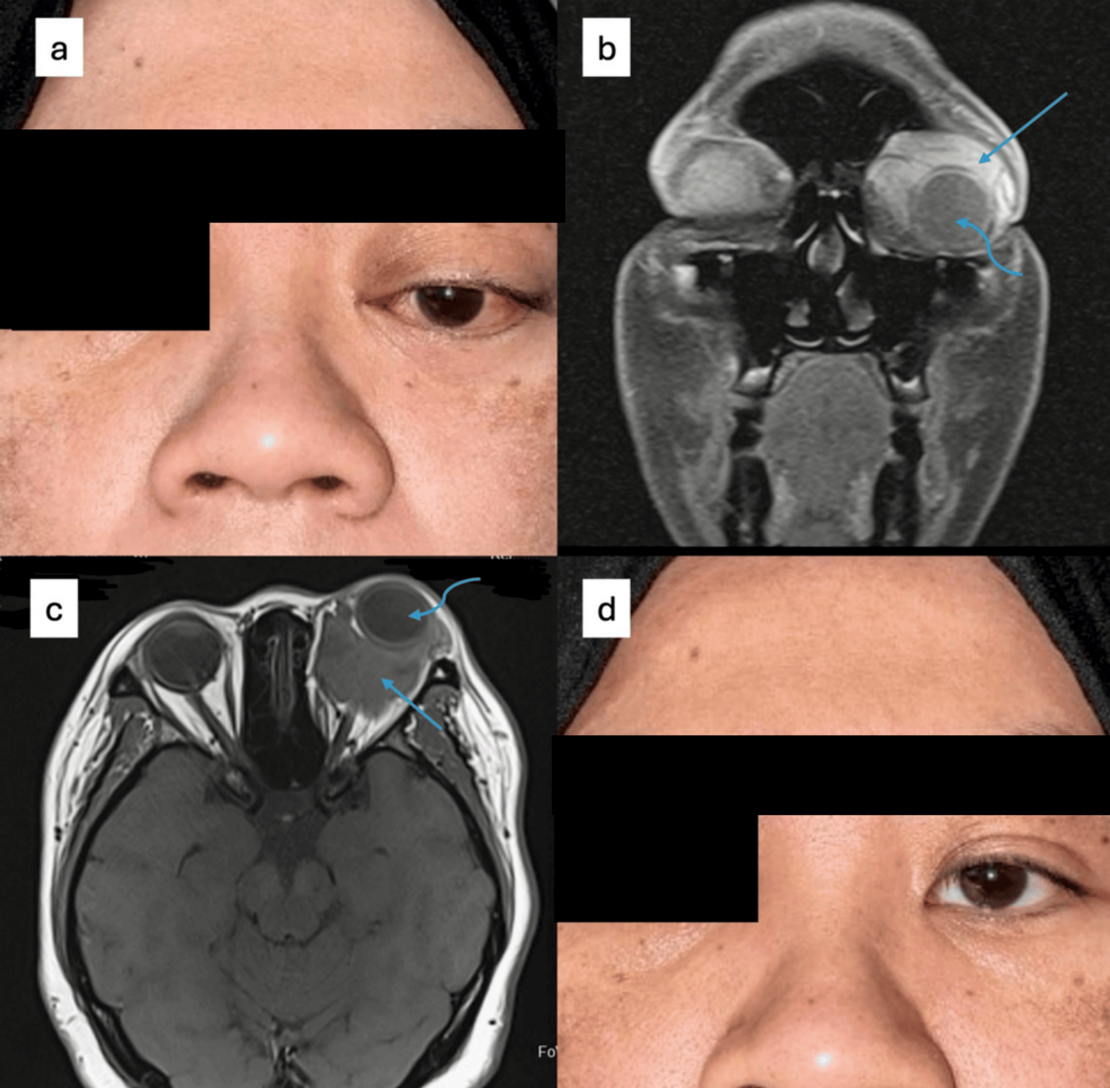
Case 2 on presentation showing proptosis and downward displacement of the left globe (a). Coronal (b) and axial (c) views of MRI T1 images show left OVLM (straight arrow) displacing the left globe (curved arrow) anteroinferiorly). An excellent response was seen one month post the second intralesional bleomycin (d). OVLM: orbital venolymphatic malformation
